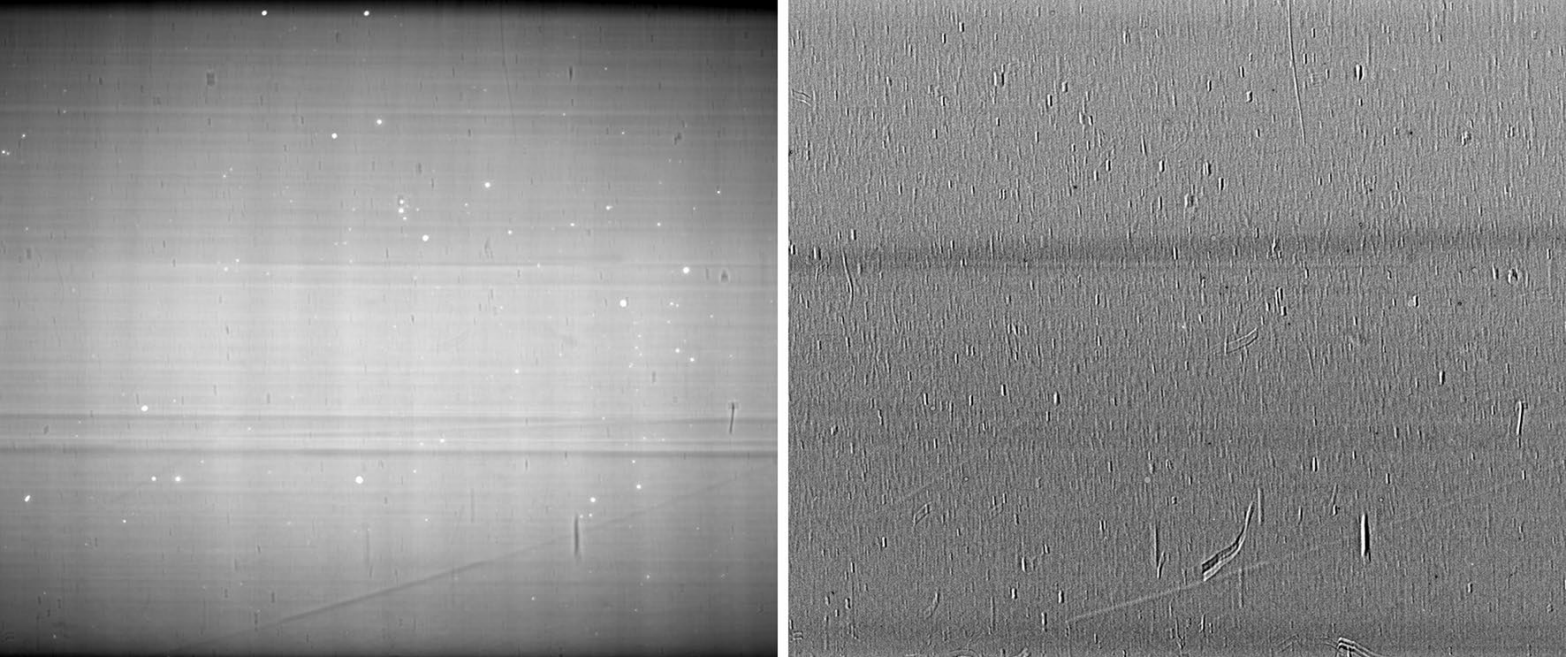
On the *left*: (average) flat field image collected before the acquisition of all the projections. On the *right*: pixel-by-pixel difference between the (average) flat field image taken before the acquisition of all the projections and the (average) flat field image collected after the full rotation of the sample (not reported). The slight variations of the flat field images due to the instabilities of the X-ray source and other beamline components (e.g., the monochromator) can be noticed
